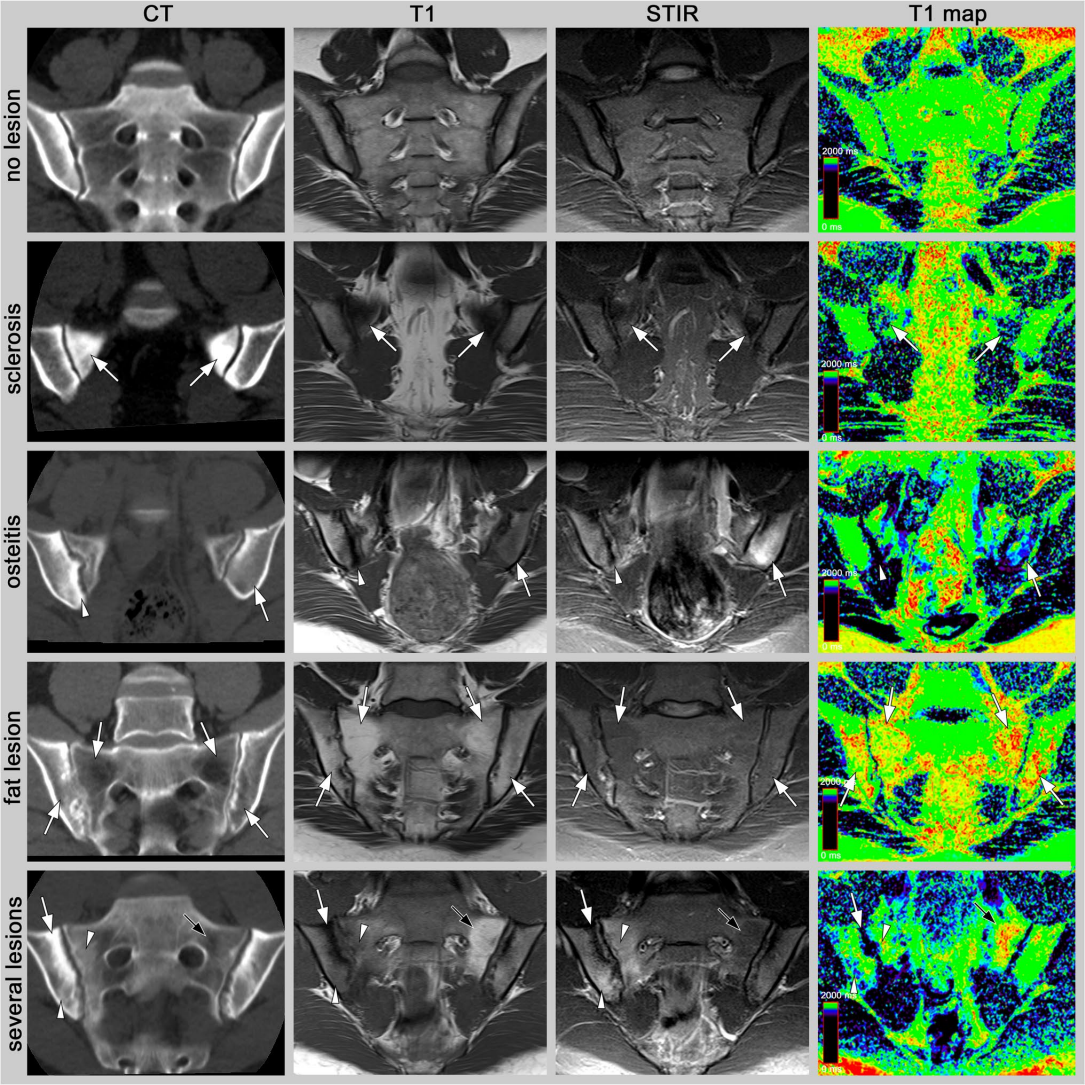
Imaging examples. Presented are different lesions in CT (window level / window width: 240/730) and MRI (T1, STIR, and T1 map). Top row: Normal appearance (no lesions). Sclerosis: A 38-year-old male patient with mechanical joint disease and prominent sclerosis in the anterior aspects of the sacral bone (arrows). Osteitis: An 18-year-old female patient with radiographic axSpA and edema (arrow) and sclerosis (arrowhead). Fat lesion: A 47-year-old male patient with advanced, inactive radiographic axSpA and bilateral fat metaplasia (arrows). Bottom row: A 21-year-old male patient with non-radiographic axSpA (mNYC grading according to radiography 1/1) and sclerosis (white arrow), bone marrow edema (arrowhead), and fat metaplasia (black arrowhead)
